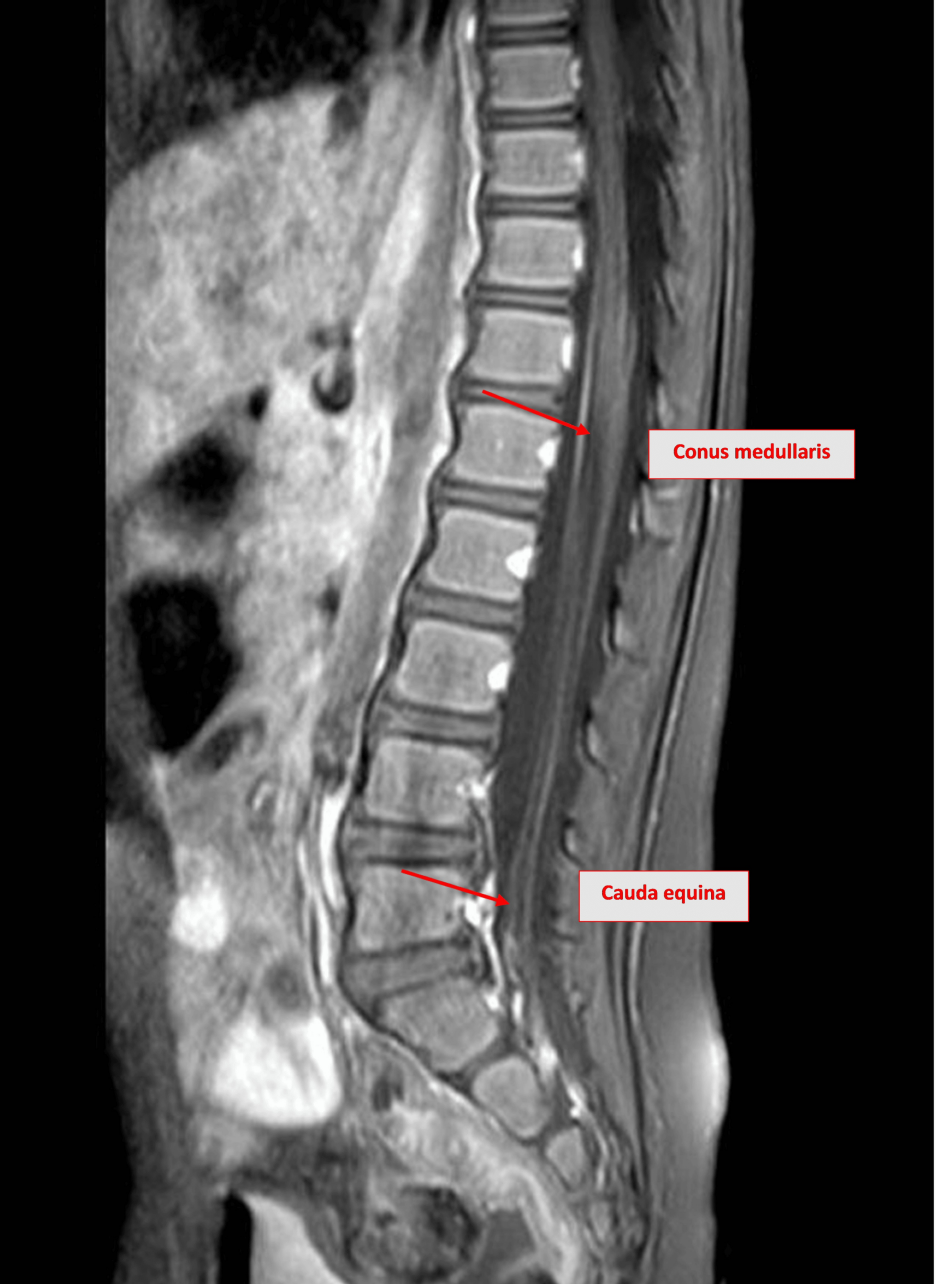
Spinal MRI of the patient on admission T1-weighted MRI of the lumbar spine demonstrating smooth thickening and enhancement of spinal nerve roots within the cauda equina and conus medullaris region (red arrows) in sagittal view, findings consistent with Guillain-Barré syndrome. MRI: magnetic resonance imaging
